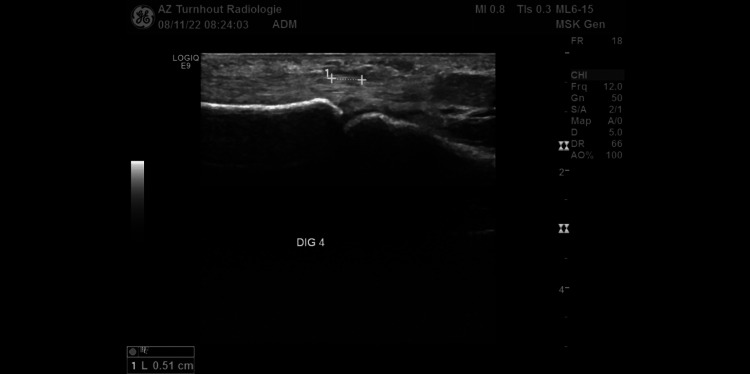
Ultrasound Investigation Flexor Digit 4 (Lesion 0.51 cm)
